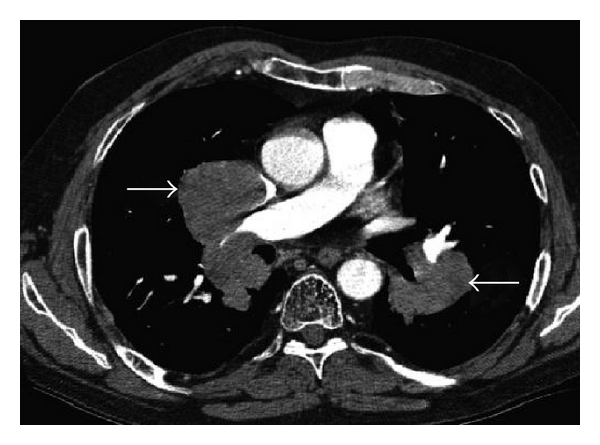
Axial CT thorax postintravenous contrast at the level of T6: diffuse bilateral hilar lymphadenopathy from biopsy-proven multiple myeloma (arrows).
